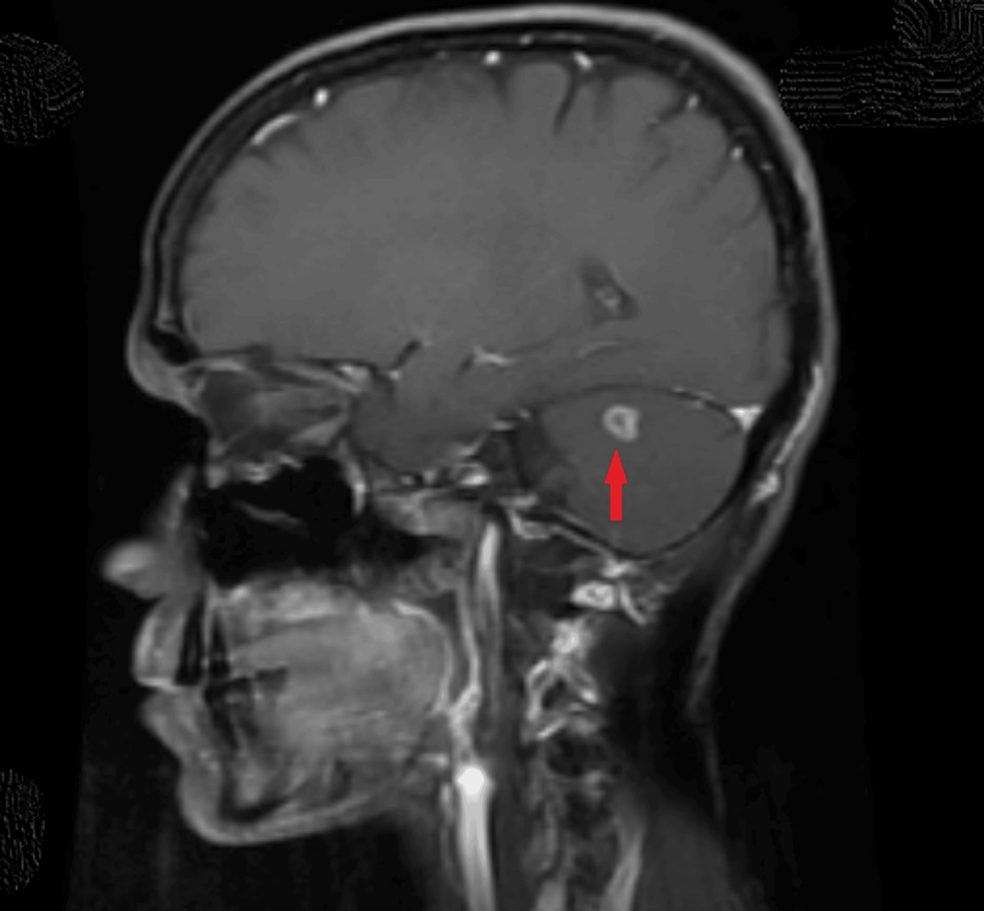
MRI of the brain: sagittal T1 section with contrast Red arrow: well-defined ring-enhancing lesion in the superior part of the right and left cerebellar hemisphere MRI: magnetic resonance imaging
